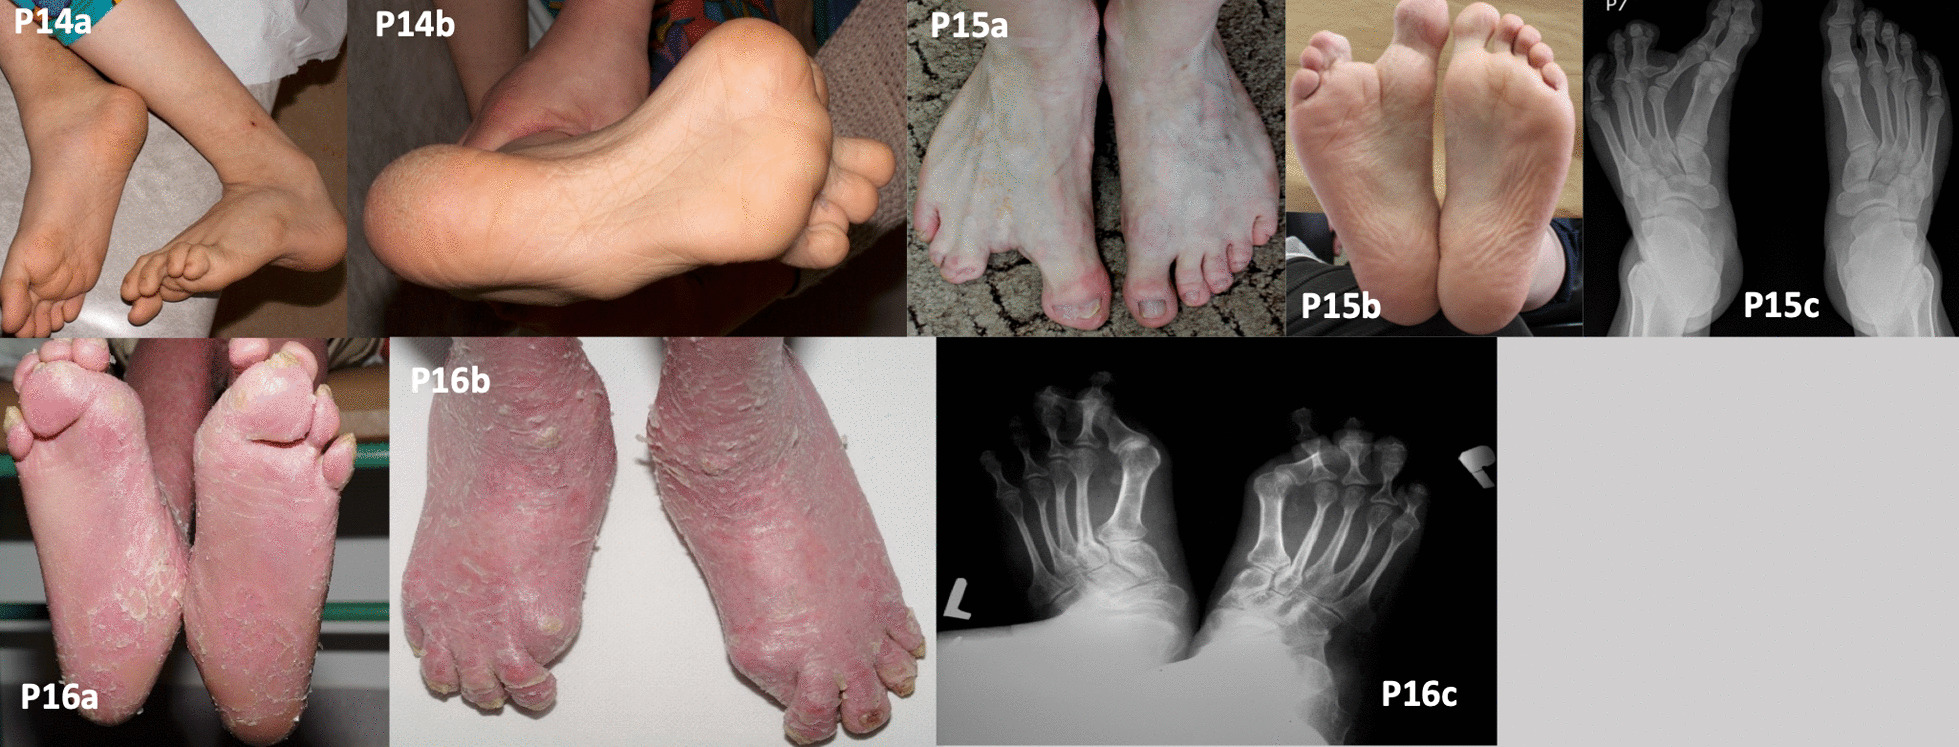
Other genodermatoses. Clubfoot in the left foot (P14a). Claw toes (P14b). Split foot deformity in a patient with ectrodactyly-ectodermal dysplasia-clefting syndrome (P15a–b). A radiograph (P15c) shows the additional residual middle phalanx of digit III in the right foot, deformed distal phalanges of digits IV and V, syndactyly digits III–IV and IV–V, a shortened middle phalanx of digit V in both feet, and the abnormal position of the distal interphalangeal joints. Hallux valgus, angling inward under toes II–IV of the right foot, and under toes II and III of the left foot, in a patient with ichthyosis with confetti (P16a–b). The same abnormalities are visible on a radiograph (P16c). Figure P16c has been previously published by Wawrzycki et al. [8] it is Open Access article distributed under the terms of the Creative Commons Attribution-NonCommercial-ShareAlike 4.0 International (CC BY-NC-SA 4.0) License
